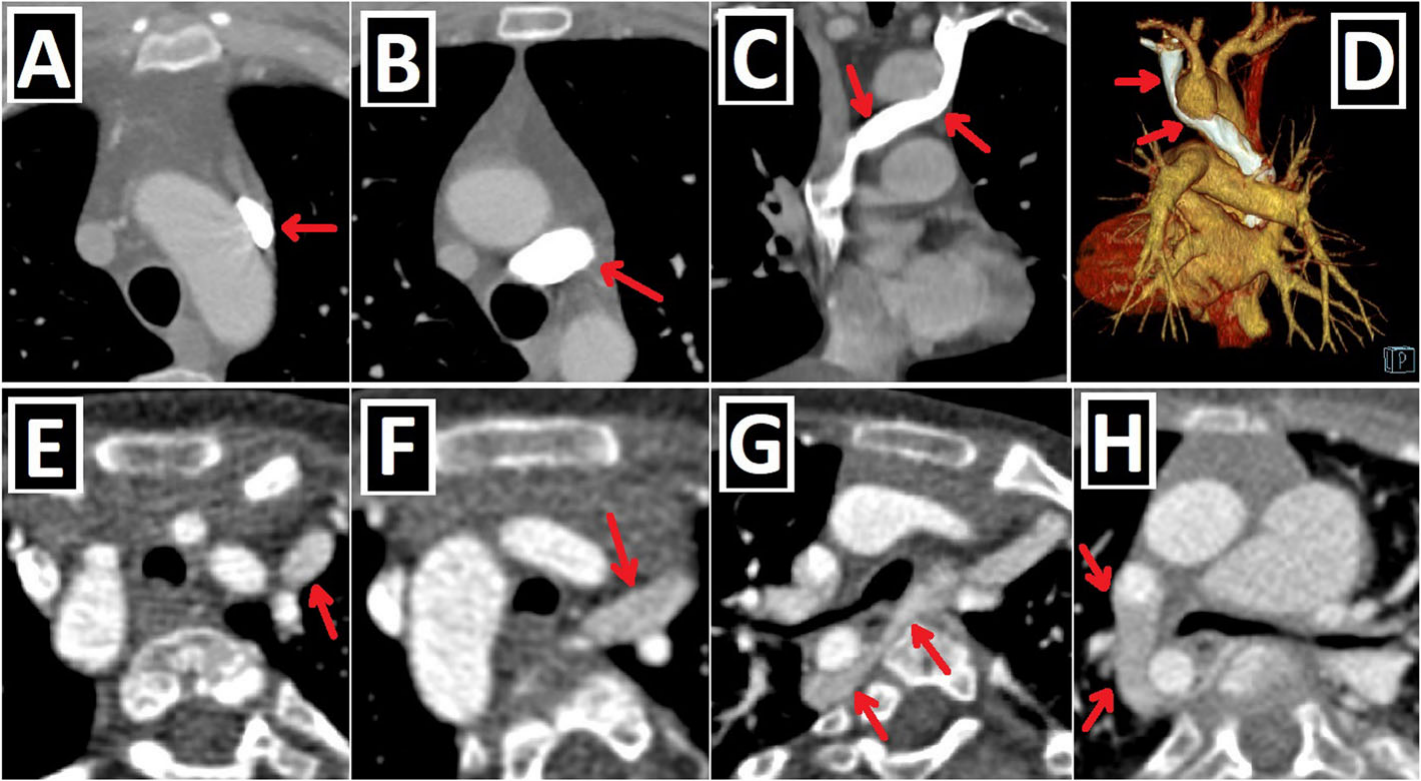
Aberrant left brachiocephalic vein. a–d Axial (a, b), coronal-oblique reformatted (c), and 3D VRT (d) CT images depict the anomalous subaortic course of the left brachiocephalic vein (red arrows). Note that no venous structure is observed in the left half of the mediastinum below the aberrant left brachiocephalic vein level. e–h Axial CT images depict an aberrant left brachiocephalic vein with an anomalous retroesophageal course (red arrows)
